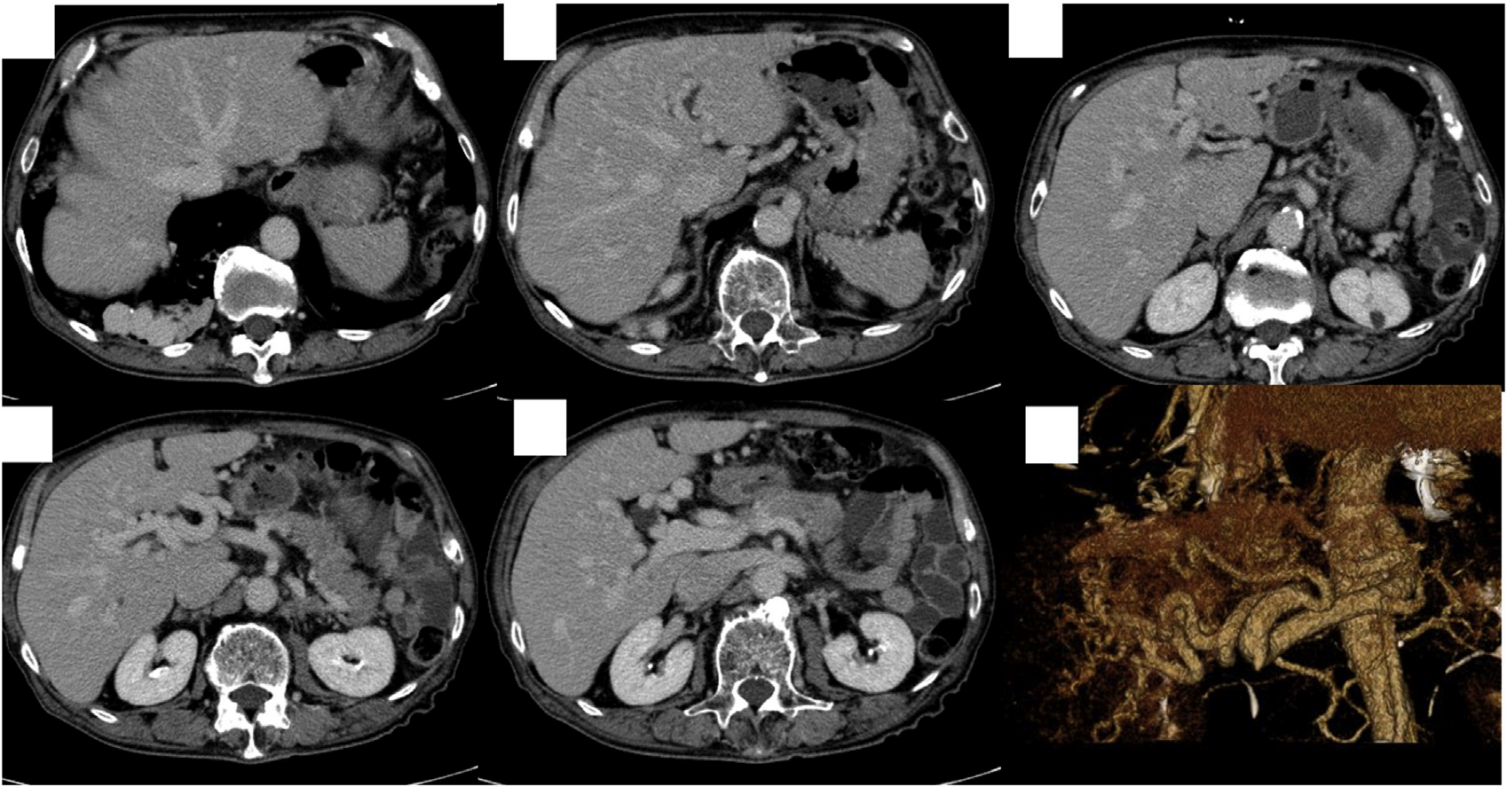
Computed Tomography of the Liver Computed tomography showed multiple arteriovenous shunts.
